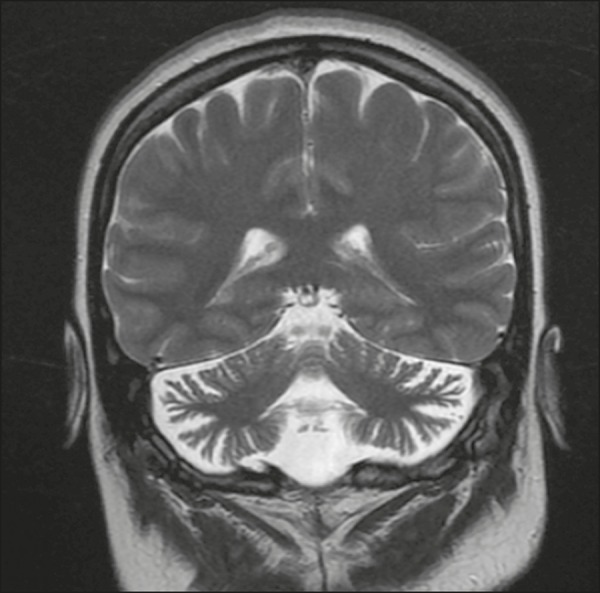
Cerebellar atrophy in a chronic phenytoin user. Coronal T2-weighted
